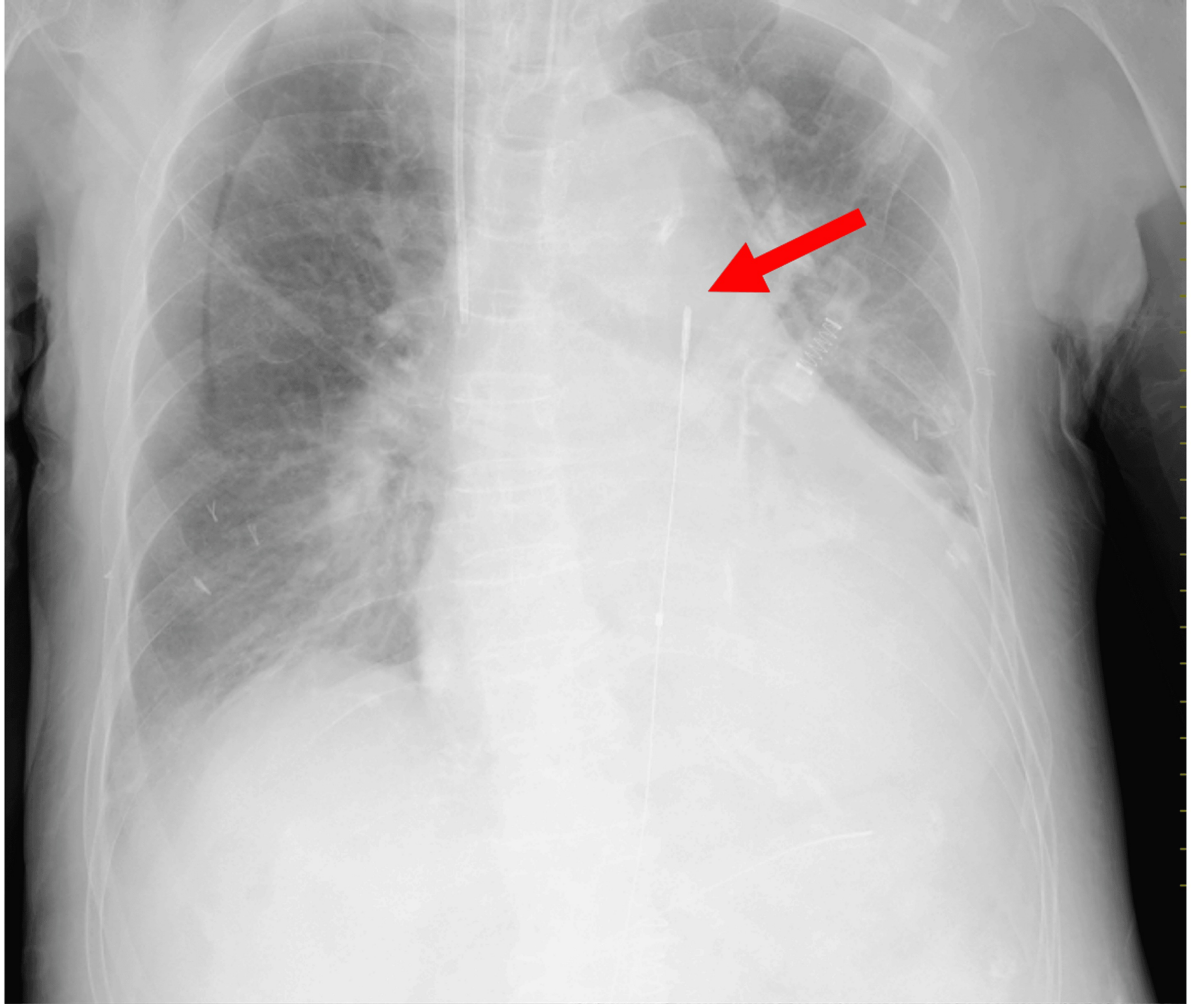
Chest X-ray demonstrating the REBOA catheter (Case 1) The red arrow indicates the tip of the REBOA catheter, which is appropriately positioned. REBOA: Resuscitative endovascular balloon occlusion of the aorta
